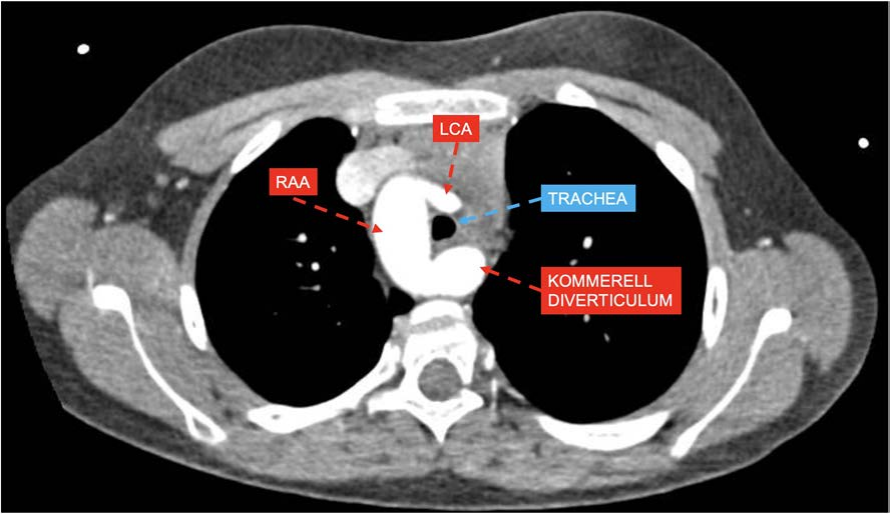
CT axial view showing right aortic arch (RAA) with aberrant left subclavian artery and Kommerell's diverticulum compressing the trachea with estimated lumen reduction of 55%; left coronary artery (LCA).
